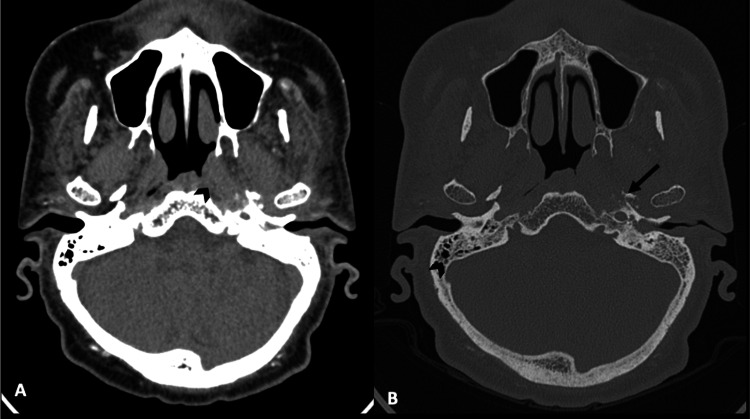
HRCT temporal (A) in soft tissue and (B) in bone windows shows a fullness of the left fossa of Rosenmuller (arrowhead) with associated erosion of the left base of the skull HRCT: high-resolution computed tomography
